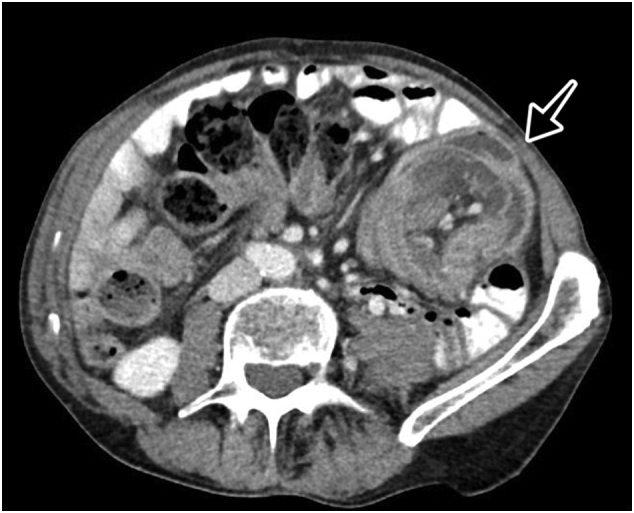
CT scan of the abdomen. Axial plane showing an ileo-colic intussusception with the classic ‘bulls-eye’ appearance (arrow).
